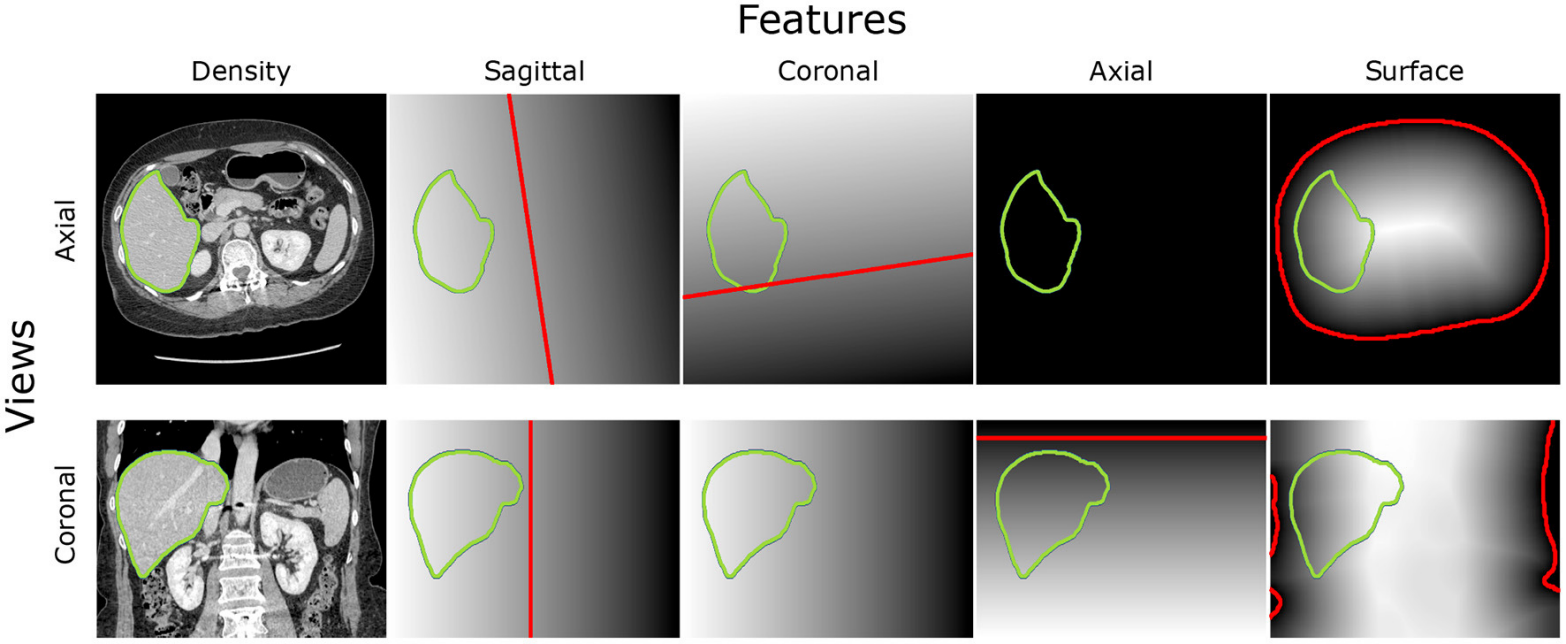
Intensity features and position features based on robustly segmentable structures: The axial view on the middle slice of the CT scan can be found in the top row, while the coronal view is in the second row. The first column is the intensity image. The other columns show the Signed Distance Field (SDF) to the sagittal plane, coronal plane, the axial plane on the bottom level of the heart, and the body surface. The zero level of the SDF is red. The border of the liver is green.
